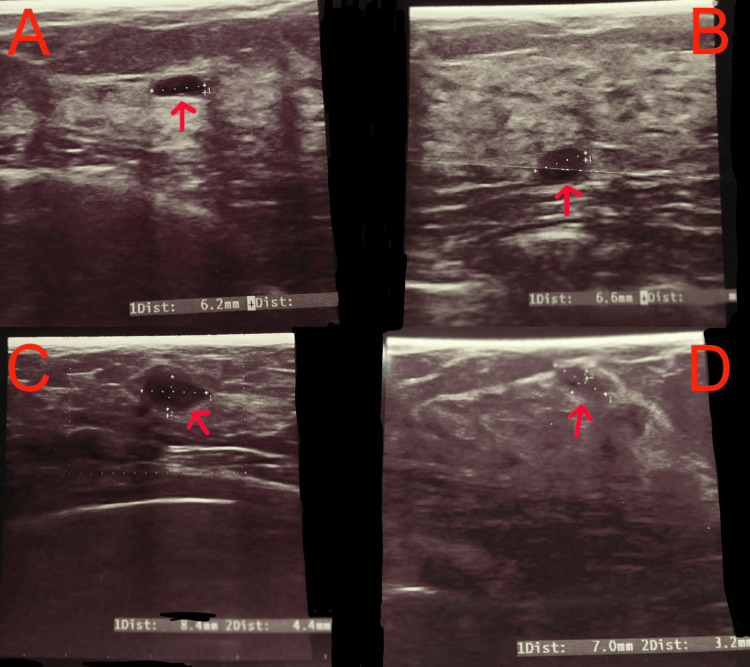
Breast ultrasound examination on November 23, 2020 A: Cyst measuring 6.2 mm in diameter (red arrow); B: Cyst measuring 6.6 mm in diameter (red arrow); C: Cyst measuring 8.4 mm in diameter (red arrow); D: Area of reduced echogenicity measuring 7×3 mm (red arrow), suggestive of a dysplastic area.
